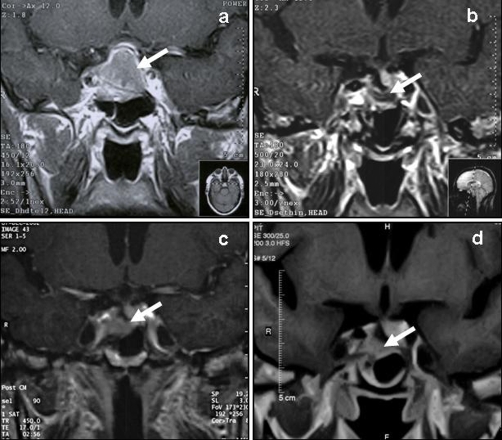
Magnetic resonance imaging scans of the pituitary macroadenoma over the years in the patient studied. Contrast-enhanced coronal T1-weighted magnetic resonance imaging showing a large pituitary macroadenoma (arrow) invading the right cavernous sinus (a). After one year of cabergoline therapy the adenoma was markedly reduced and the intrasellar remnant (arrow) was modest (b). After two years of therapy a minimal, but evident, increase in the intrasellar remnant (arrow) was observed (c). Two years after stereotactic radiosurgery the intrasellar remnant (arrow) was stable (d).
